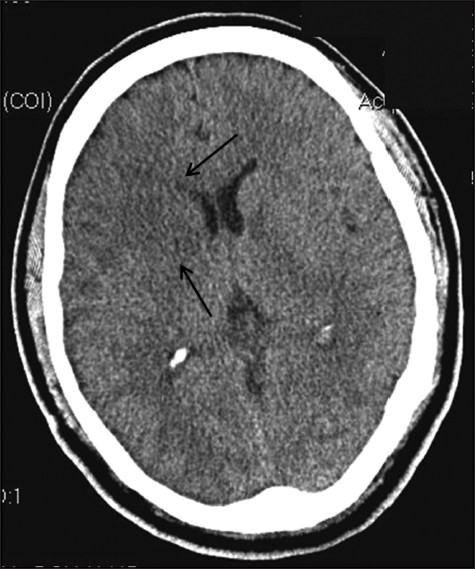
Axial CT scan of the brain without contrast 2 hours after completion of prostatectomy demonstrates early lucency of evolving infarction in the right MCA territory
